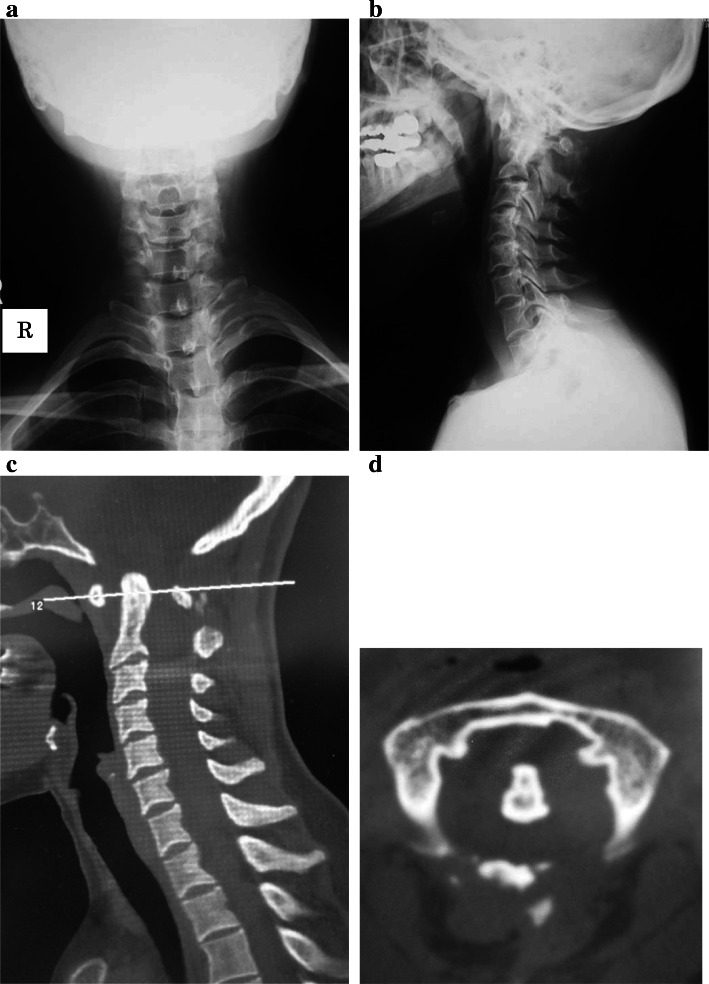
Recurrence of atlanto-axial dislocation. a Anterior–posterior radiography image. b Sagittal radiography imaging. The upper atlantodental interval was 8.5 mm. c Computed tomography sagittal image showing the recurrence of the atlanto-axial dislocation. d Computed tomography axial image showing the fracture of the C1 lamina
